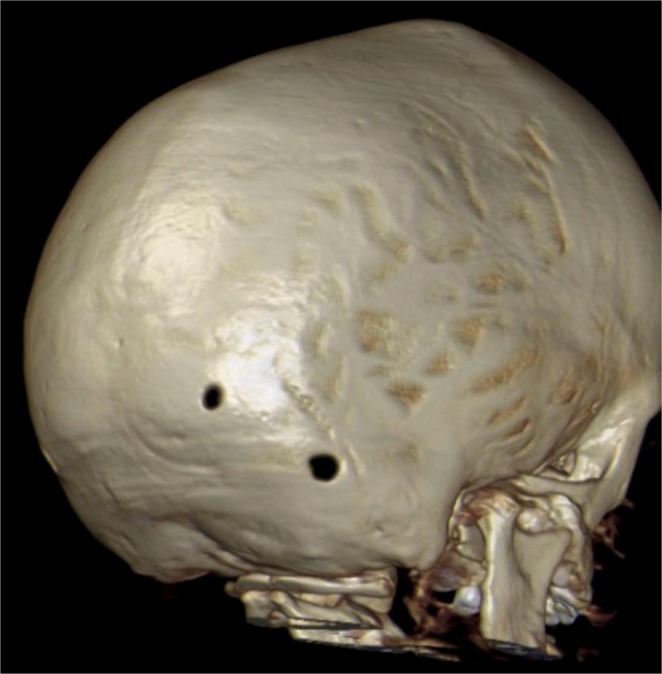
3D CT reconstruction of a child with pansynostosis and found to have giant foramina located in the occipital bone, which indicate larger than normal occipital emissary veins traversing the occiput and a potential hazard with any cranial surgery in this region
